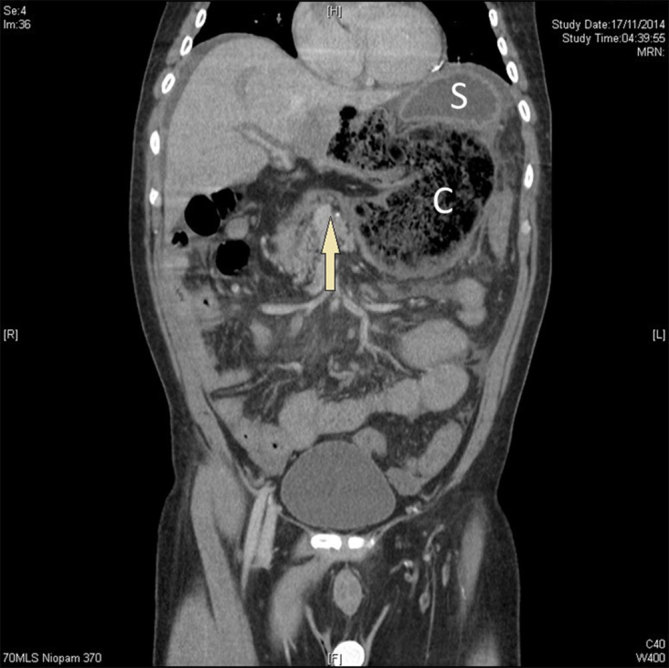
A coronal section of the CT scan showing the caecum (C) herniating through the foramen of Winslow (arrow), lying below the stomach (S).
